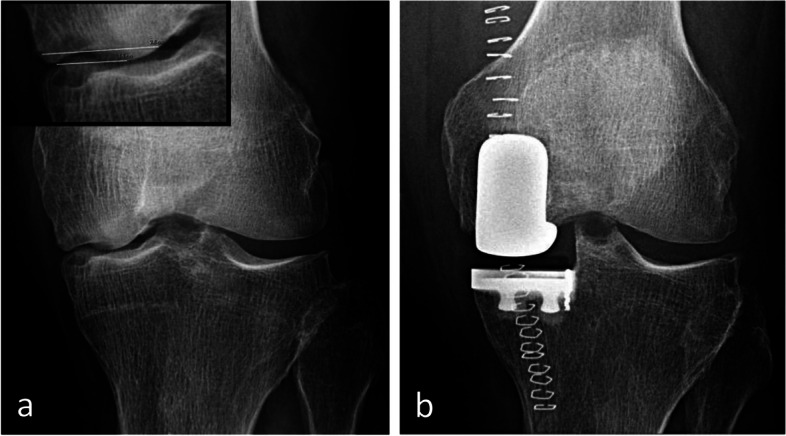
Evolution of subchondral osteonecrosis related to insufficiency fracture (SIF-ON) according to the size of the necrosis. a Radiograph of this SIF-ON shows a collapse of the inferior pole of a condyle, which extends to more than 50% of its width (64%). Its pejorative evolution led to the placement of prosthesis a few months later (b)
